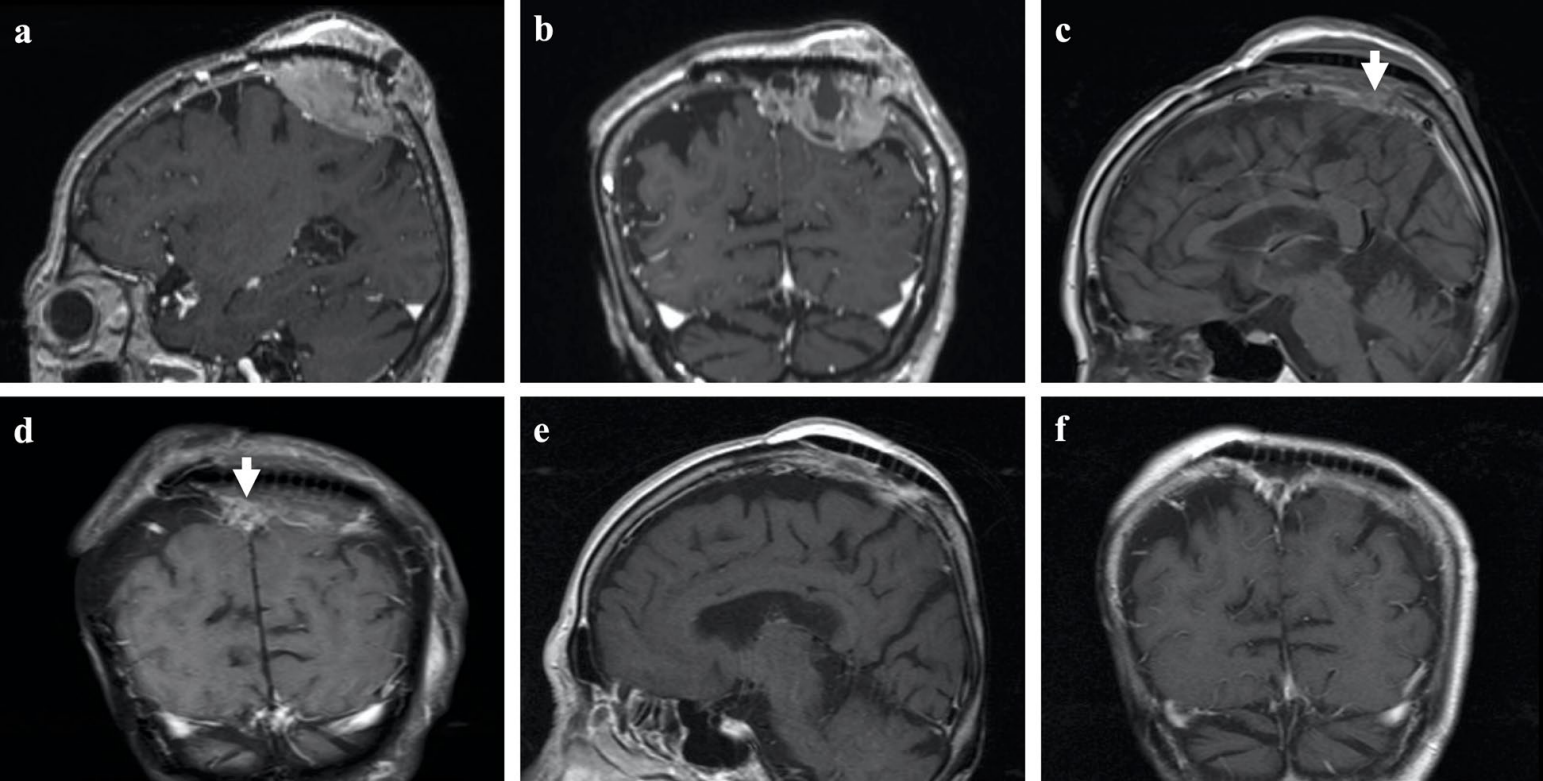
Magnetic resonance imaging before and after third surgery. A heterogeneous, gadolinium-enhancing tumor is observed in the epidural region with extracranial extension pushing against the titanium mesh. The SSS is nearly compressed but is not occluded, and the scalp is about to rupture (a sagittal view; b coronal view). The recurrent tumor is resected with the skin flap, titanium mesh and surrounding bone edge. Tumor invading the SSS is not resected (arrows). An artificial bone flap made from polyethylene is used for cranioplasty. Scalp reconstruction is made with a new pedicled skin flap (c sagittal view; d coronal view). The residual tumor shows rapid shrinkage and has vanished by 3 months after radiotherapy (e sagittal view; f coronal view)
